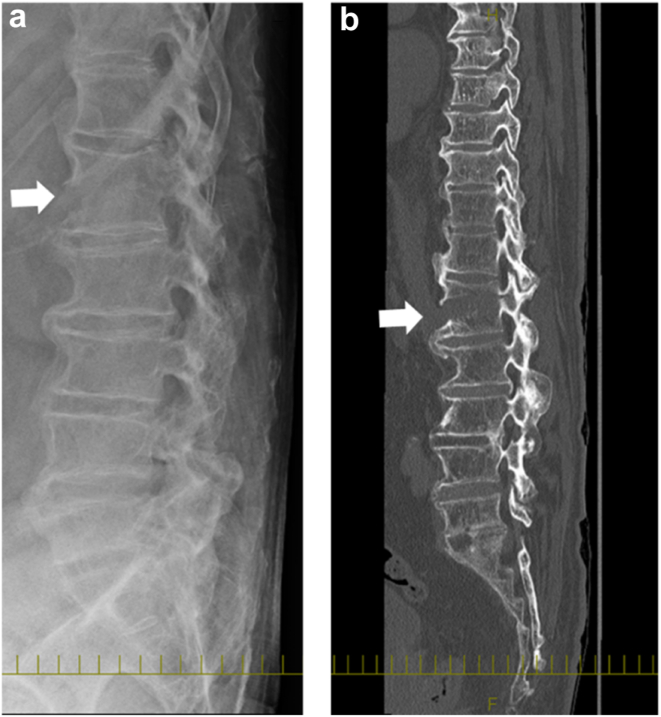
Postoperative spine radiograph and CT. (a) Lateral view of radiograph and (b) Lateral view of CT. White arrows indicate the fracture line extending to the posterior column.
